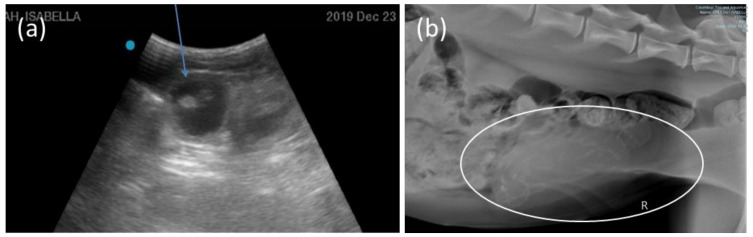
Pregnancy monitoring of recipient female by ultrasonography 32 days post-transfer, the arrow is pointing at the embryonic vesicle filled with fluid (a) and radiography 62 days post-transfer, mineralized skeletons of both cubs are visible within the circle (b).
